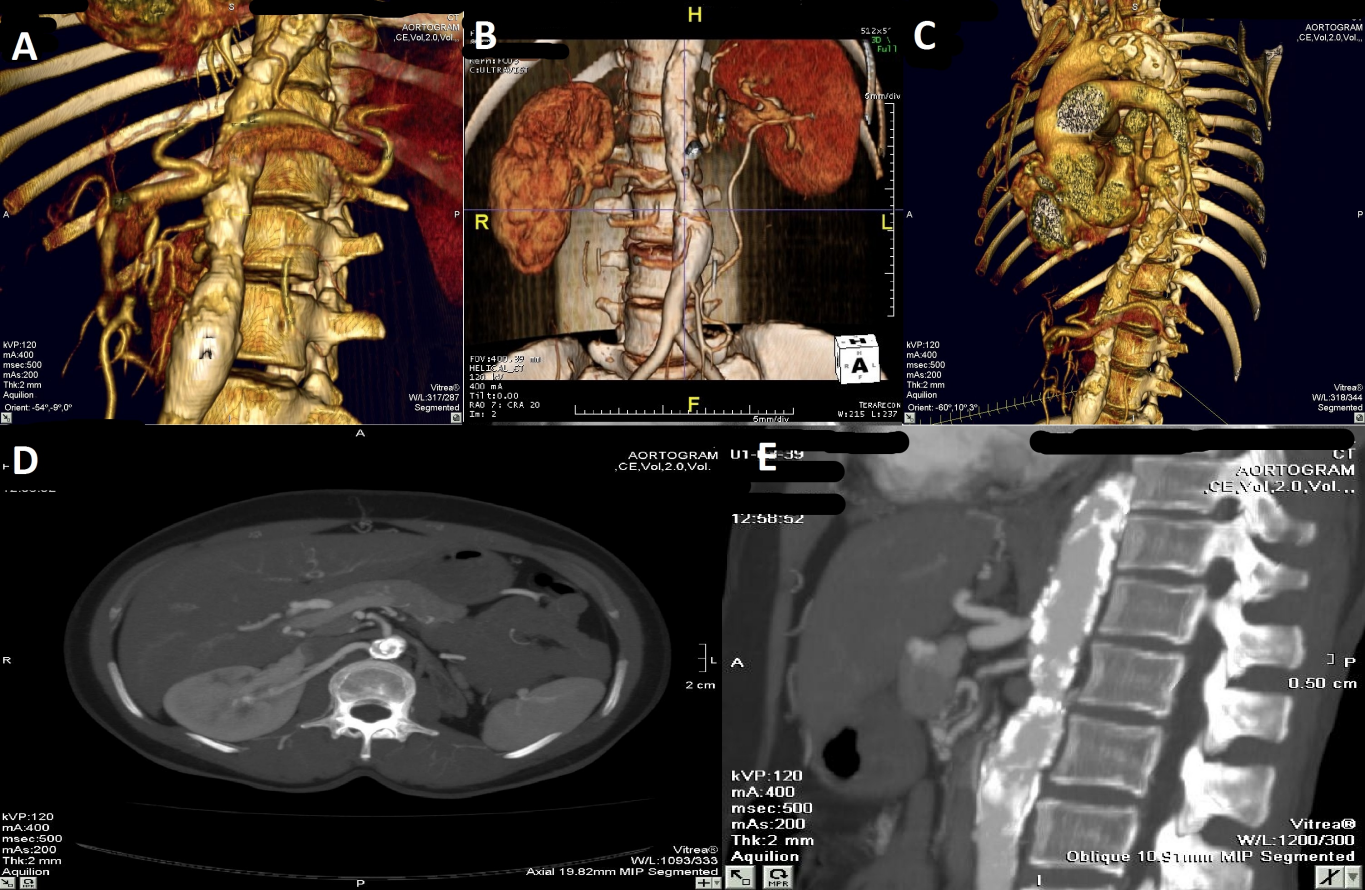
Computed tomography (CT) aortogram of abdomen [A-E] Occluded left renal artery while right renal artery is normal. The abdominal aorta is mildly narrowed at and below the level of renal arteries.
